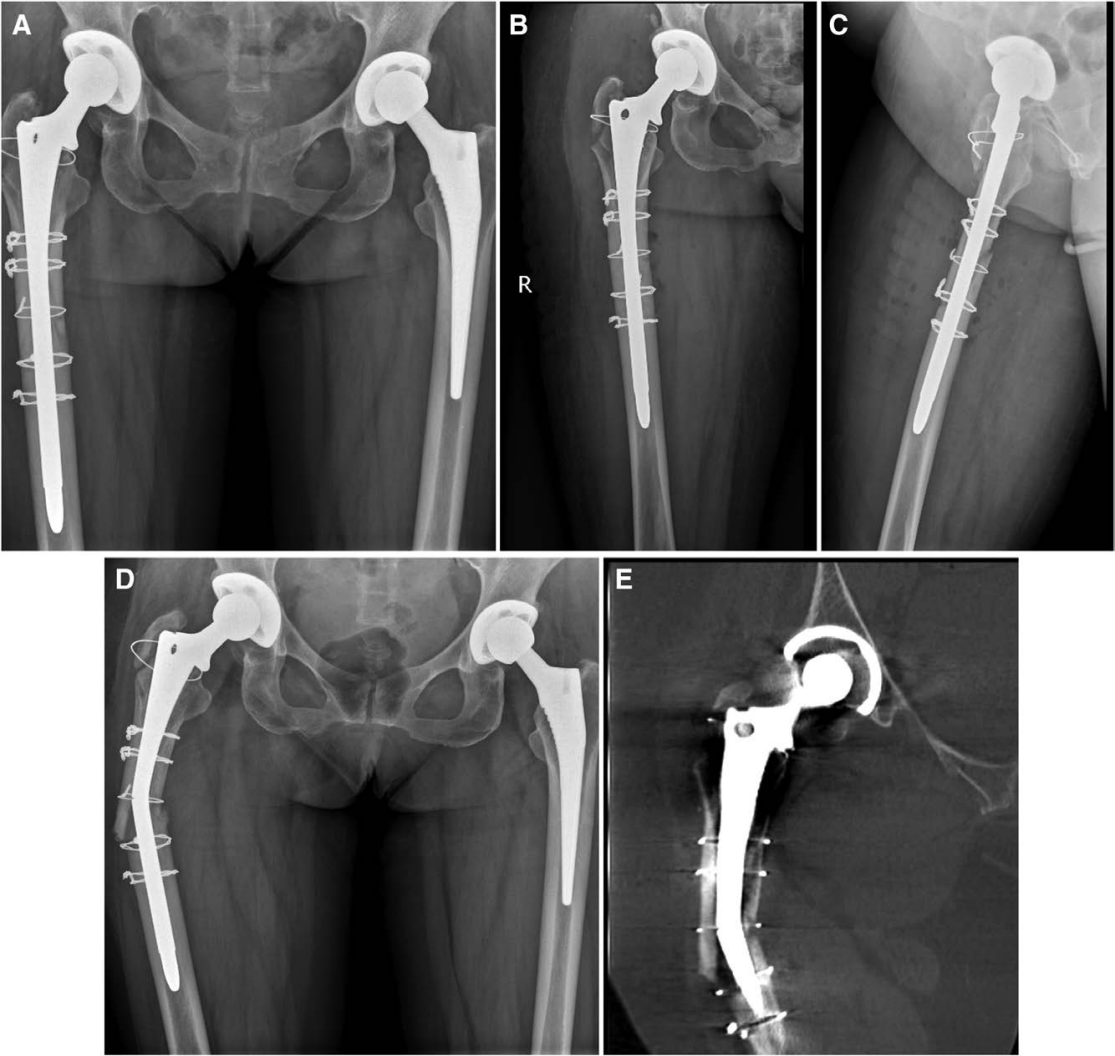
Radiographic analysis after the first revision of hip joint and entering the hospital this time. A–C: Orthostatic radiography of both hips after the revision of the right hip joint; anterior lateral radiography of the right hip; D, E: Orthostatic radiography and CT of the right upper femur fracture after the revision of the right hip joint.
